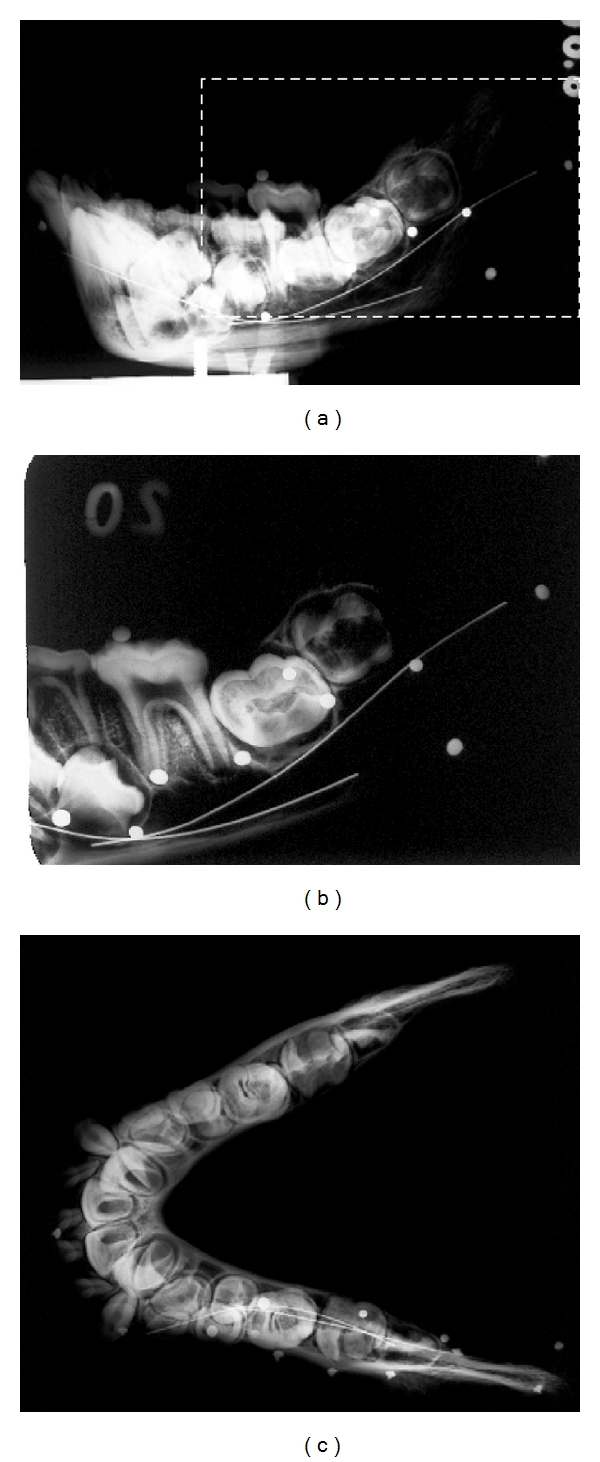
Example of radiographic image data used to determine approximate relative dental age (ARDA): *Pan troglodytes* mandible in lateral (a), intraoral (b) and dashed box in panel (a), and occlusal views.
